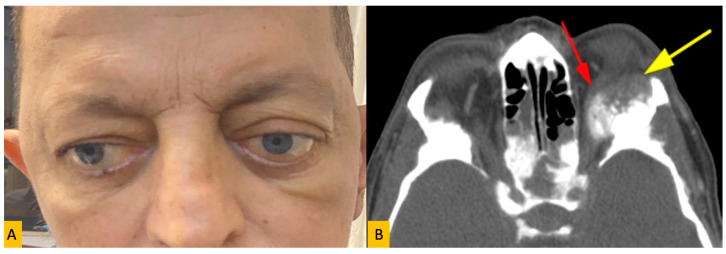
(A) Clinical photograph of a 49-year-old man with orbital metastasis of prostate cancer showing proptosis in the form of ptosis of the left eye. (B) The orbital computed tomography axial section shows a metastatic soft tissue lesion in the left orbital roof extending into the orbita and placing pressure on the superior rectus muscle (red and yellow arrows).
